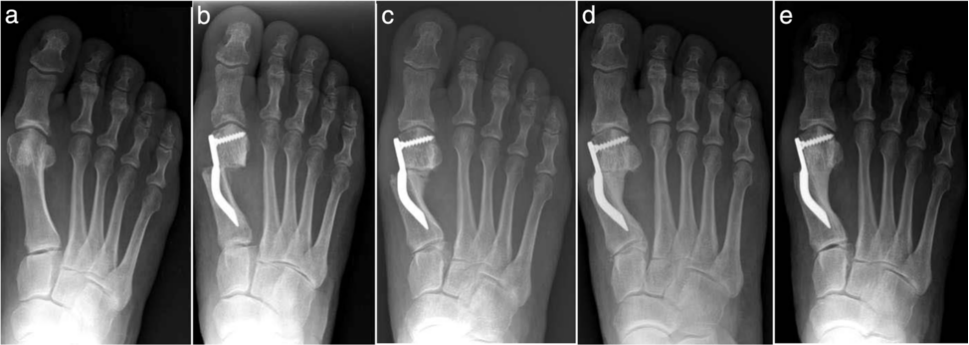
Case 3. A 57-year-old woman with mild HV; antero-posterior radiographic images at: a Preoperative period. b 1-month follow-up. c 3-month follow-up. d 6-month follow-up. e 48-month follow-up showing the maintained correction of the deformity
